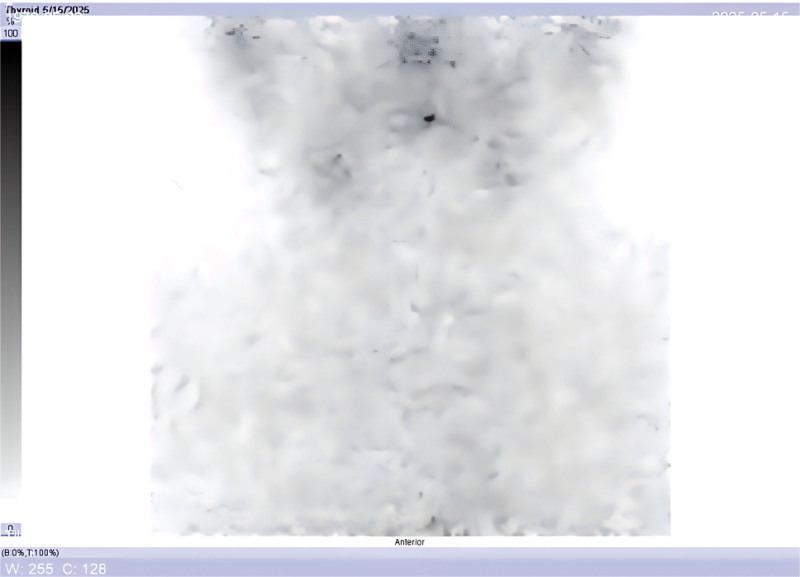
^99m^Tc-pertechnetate thyroid scintigraphy performed one month after levothyroxine withdrawal (endogenous TSH stimulation) shows no abnormal uptake in the anterior neck. TSH = thyroid stimulating hormone.
